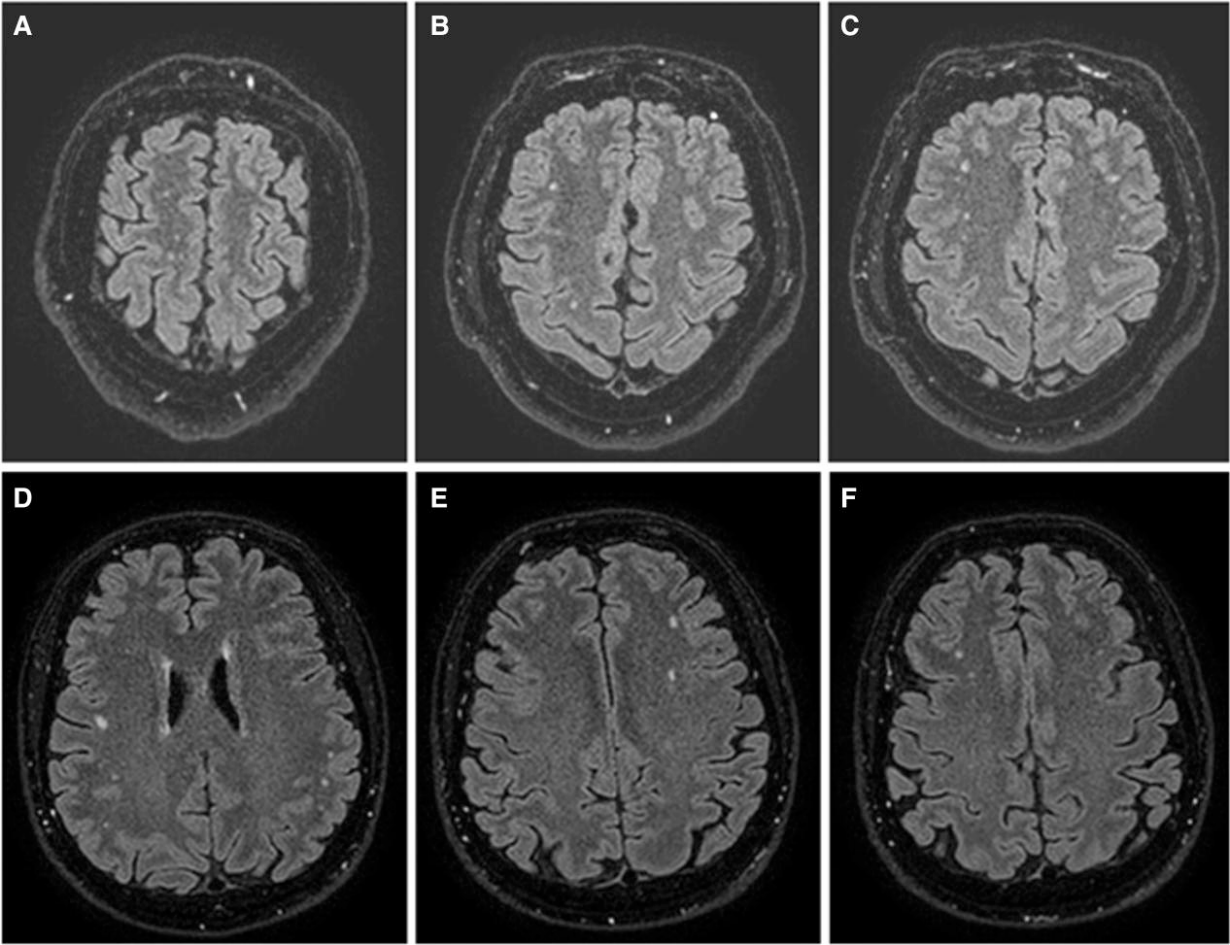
FLAIR images in two different representative participants with mild COVID-19. FLAIR images 9 and 4 months after mild COVID-19 symptom onset, respectively, (A–C) in a 46-year-old man depicting multiple nonconfluent multifocal WMHs on FLAIR images, rounded/ovoid shape, mostly located in the juxtacortical frontoparietal bilateral WH, without any mass effect nor diffusion restriction and (D–F) in a 61-year-old man showing similar findings to the previous, adding some other locations for the hyperintensity on FLAIR images, such as the left centrum semiovale.
